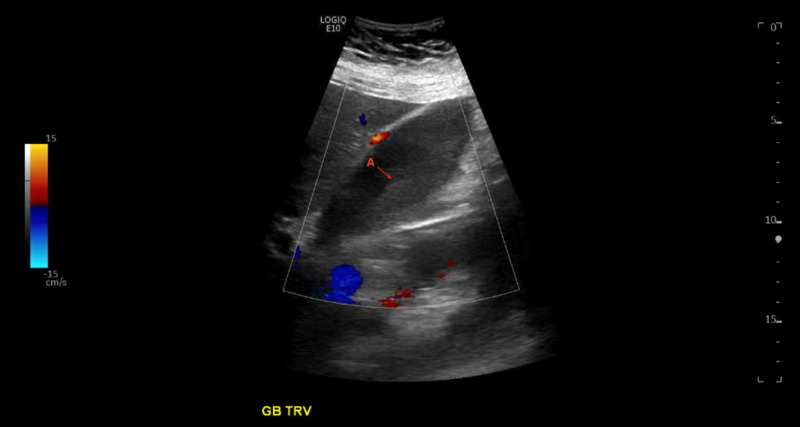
Gallbladder ultrasound. Ultrasound of the gallbladder showing biliary sludge (A)
